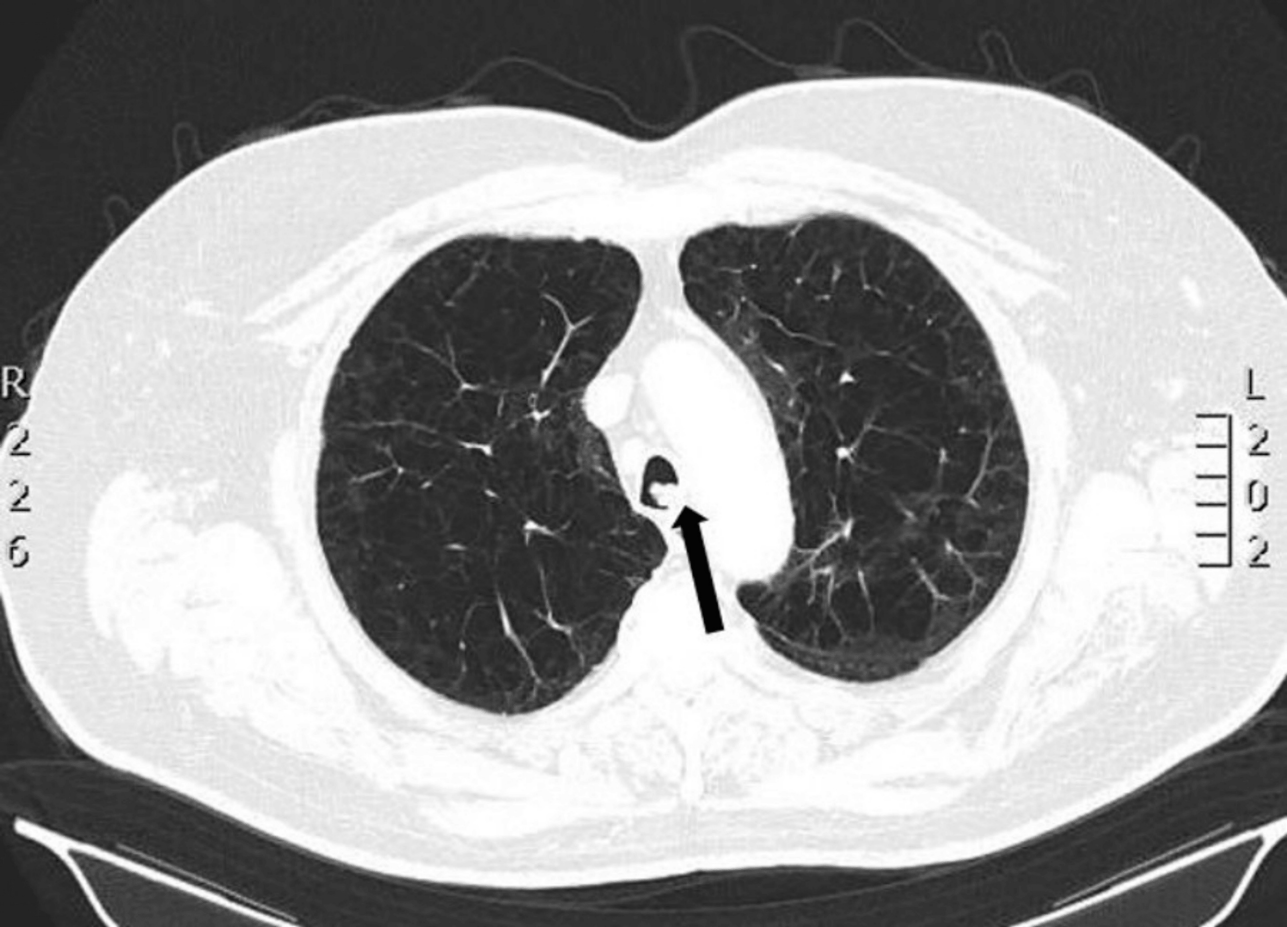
Chest CT (lung window) The image showed papillomas (black arrow) on the left wall of the trachea and significant emphysema in the lung parenchyma CT: computed tomography
